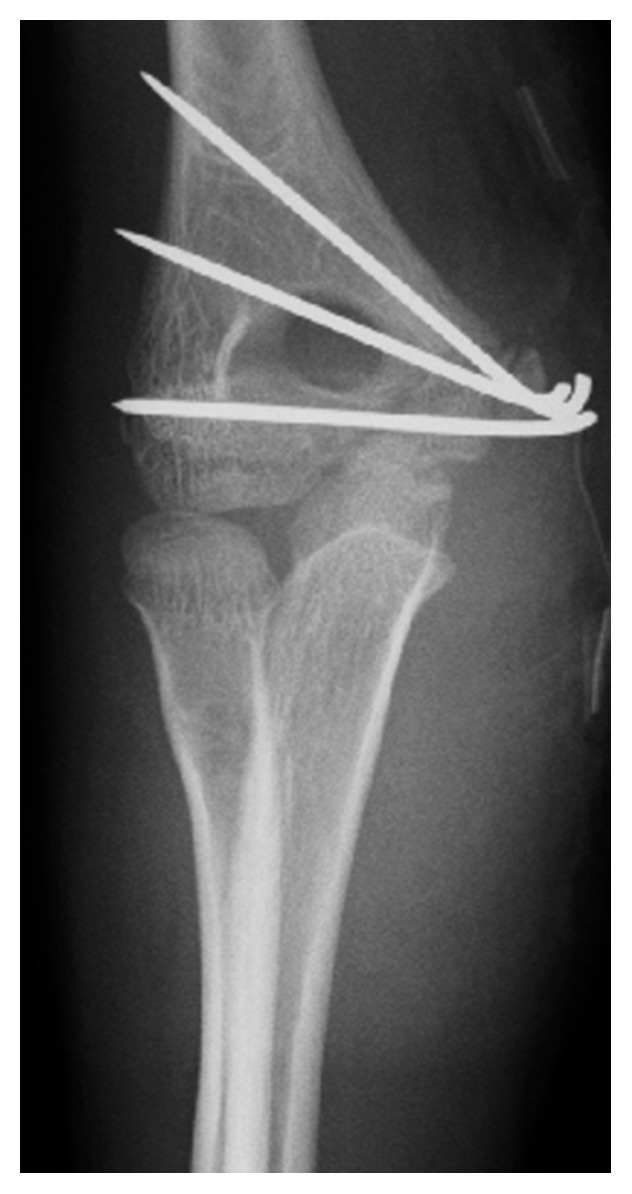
Postoperative anteroposterior radiography. The bone fragment was fixed in an accurate position with three smooth Kirschner wires.
